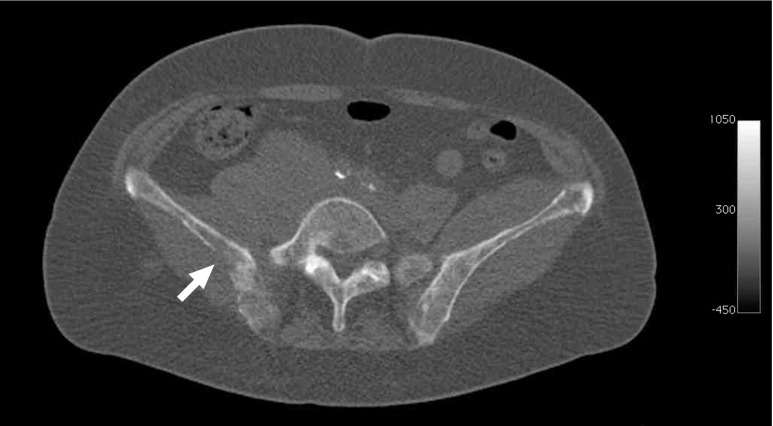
Example of tumor-induced cortical destruction at CT in a 69-year-old woman with newly diagnosed DLBCL. Axial CT shows pathological densities in both iliac wings, indicating lymphomatous bone marrow involvement, and a focal disruption of the bony cortex (*arrow*)
